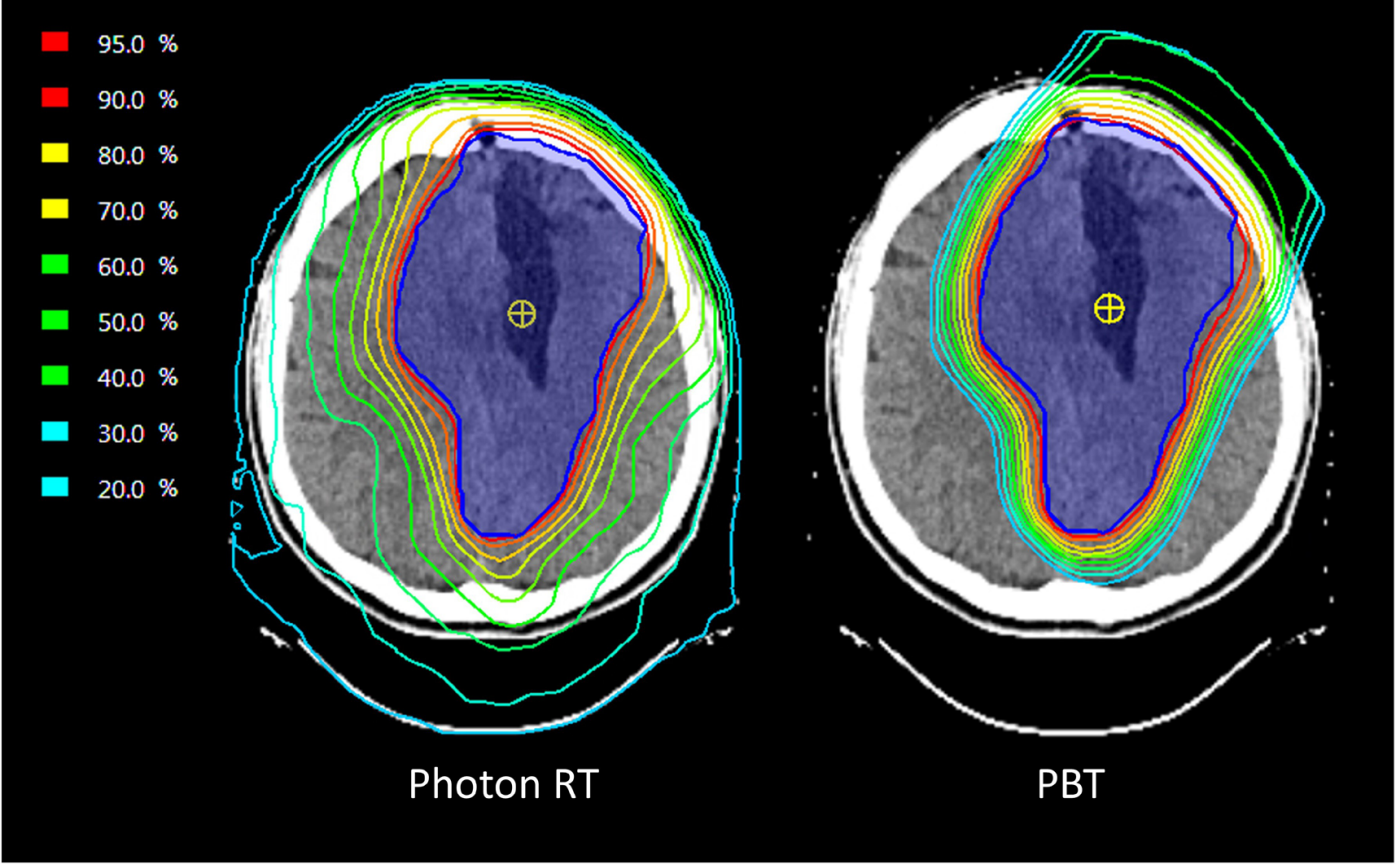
Photon RT and PBT radiotherapy dose distributions in a patient treated for ODG. Planning CT axial images of the brain showing radiation dose distributions for corresponding photon RT and PBT plans. In each plan, the dark blue contour represents the Planning Target Volume (PTV). The isodose lines represent the radiation dose to be delivered as a percentage of the prescription dose, as indicated in the key; warmer colours/higher percentages represent regions of higher dose. Greater dose sparing of normal brain structures outside of the PTV is apparent in the PBT plan. It is currently uncertain if this translates into a clinical improvement in neurocognitive function. ODG, oligodendroglioma; PBT, proton beam therapy; RT, radiotherapy.
